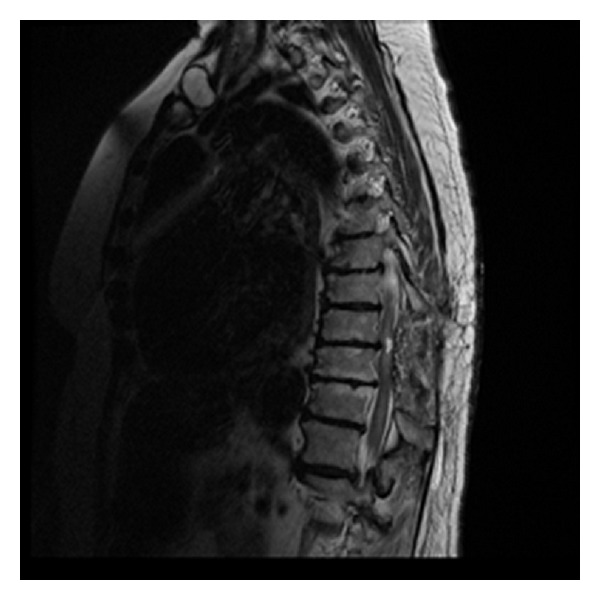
Postoperative sagittal thoracic MRI demonstrated total resection of ISCM.
